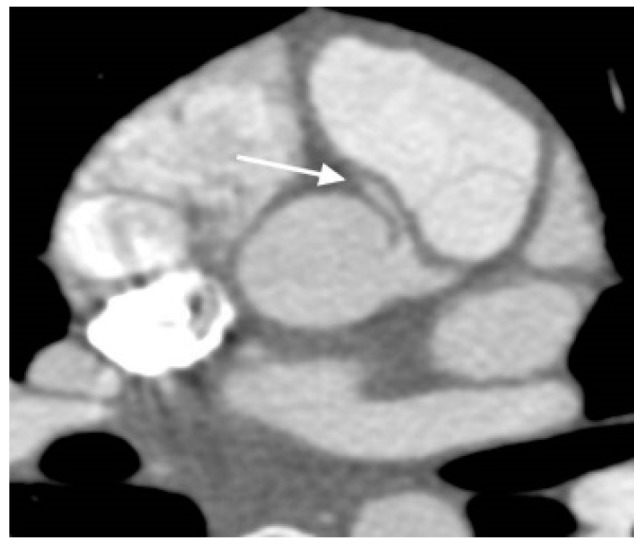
Axial Cardiac Computed Tomography Angiography (CCTA) image demonstrates anomalous right coronary artery origin from the left sinus of Valsalva with intra-arterial course (arrow).
